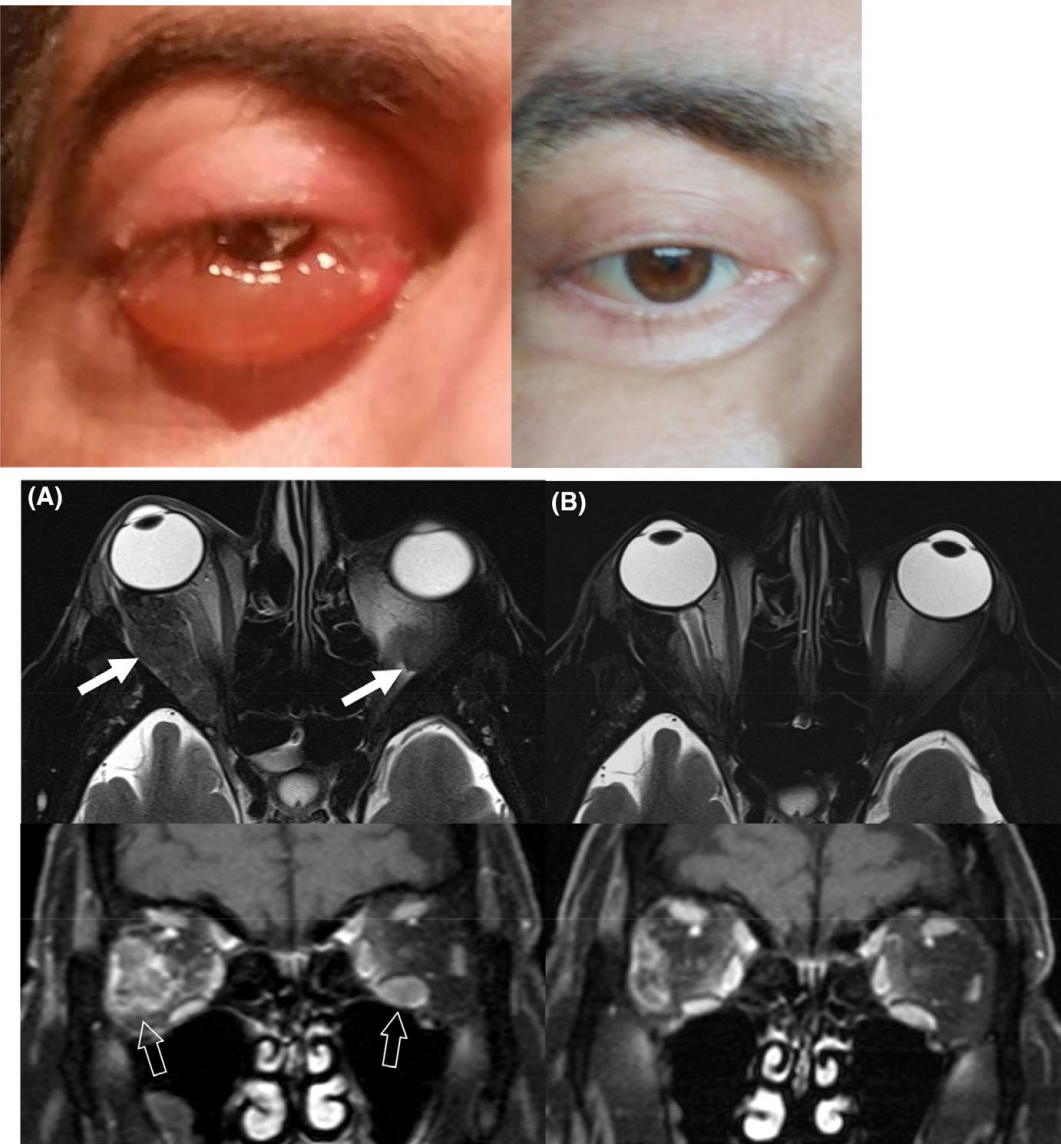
Top left: Patient eye before therapy; A, bottom: orbital magnetic resonance imaging shows disease involvement of right lateral (*closed arrows*) and left inferior (*open arrows)* recti muscles which showed increased thickness; A, upper row and lower row: low signal intensity on fat‐suppressed T2‐weighted axial images and inhomogeneous contrast enhancement on gadolinium‐enhanced fat‐suppressed T1‐weighted coronal images; top right: clinical improvement after therapy; B, bottom, upper row and lower row: orbital MRI 1 mo later fat‐suppressed T2‐weighted and gadolinium‐enhanced fat‐suppressed images, respectively, showed a clearcut improvement in muscular thickness resulting in reduction of bilateral exophthalmos
